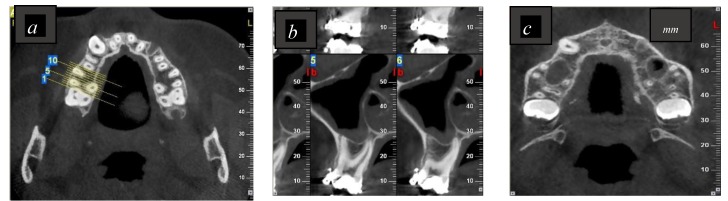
Representative CBCT images showing the palatal root apex in contact with the MSF. (a,c) A maxillary axial CBCT view; (b) a maxillary sagittal CBCT view. 1; 5 and 10 are the slices numbers.
